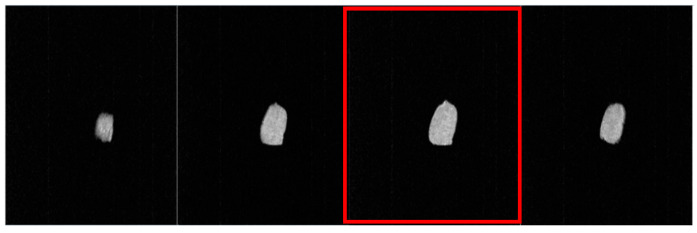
Original image of T2-weighted image.
